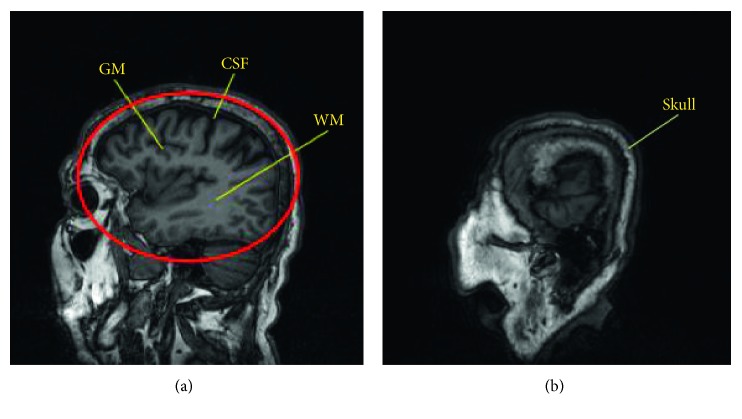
MRI images: (a) location of the 3 tissues. WM is the area where the color is light; GM is the gray boundary around the WM; CSF is the black parts inside the skull. We focus on segmenting the tissues in the red circle. (b) Cross section of a side of the brain; the tissues are hard to distinguish by eyes.
